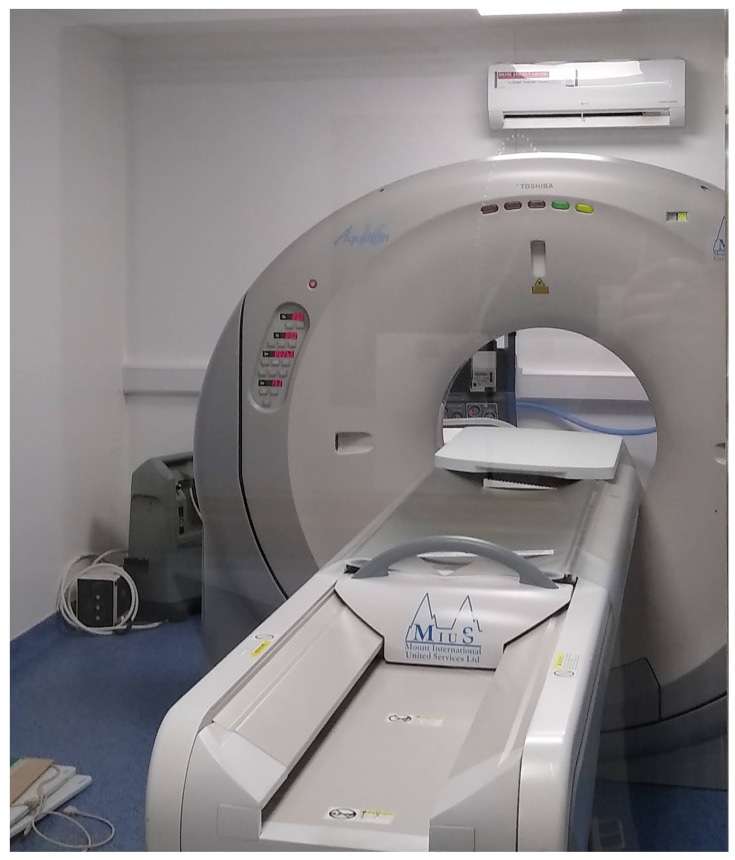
A traditional medical Toshiba Aquilion CT was used to image the coil configurations.
